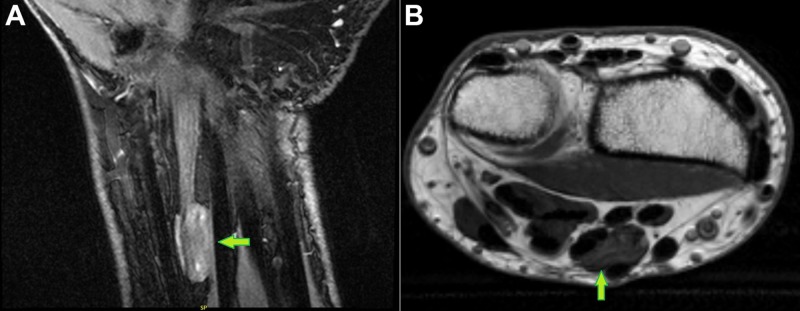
Magnetic Resonance Imaging of the Wrist Green arrows point to the median nerve mass: A) coronal view; B) axial view
